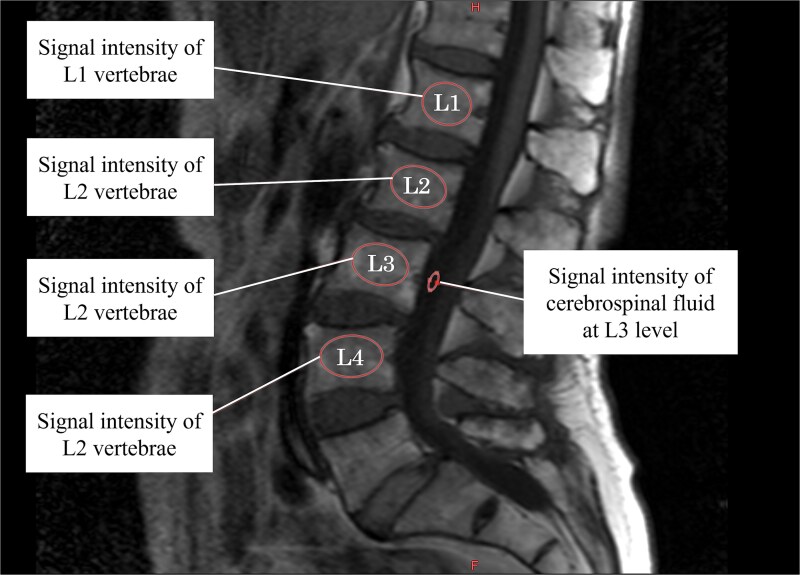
Measurement of the vertebral bone quality (VBQ) score. The VBQ score was measured on mid-sagittal T1-weighted images of lumbar spine magnetic resonance imaging (MRI). The VBQ score was calculated by dividing the average signal intensity within the vertebrae from L1 to L4 by the signal intensity of cerebrospinal fluid at the level of L3.
